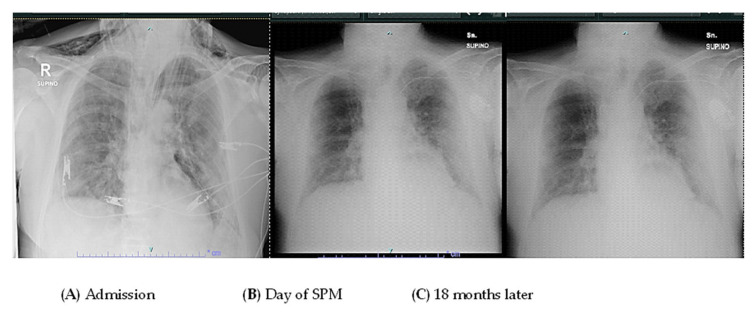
The figure reports a chest radiograph of the patient at admission (A) at the day of the SPM (B) and 18 months later (C).
